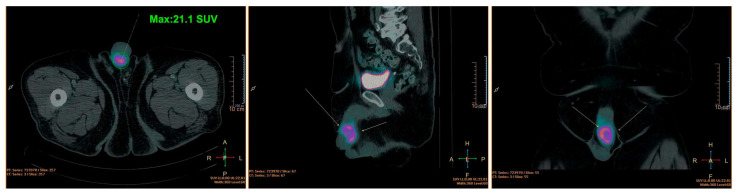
PET-CT showing the presence of an anomalous concentration area due to the lesion in the penile body. The colors indicate an anomalous concentration area of 18F-DG and correspond to a site of abnormal local cellular metabolic activity (active lesion).
